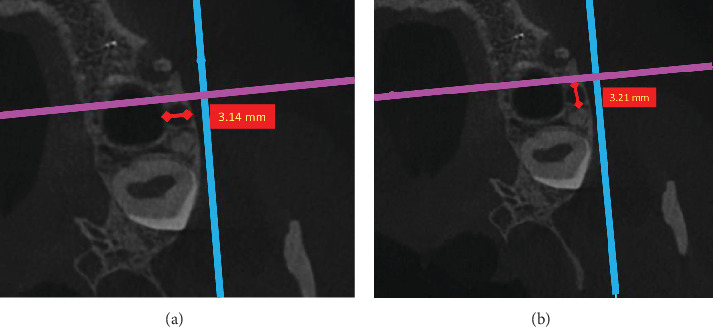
Periapical lesion extension in axial view: (a) buccolingual dimension and (b) mesiodistal dimension.
